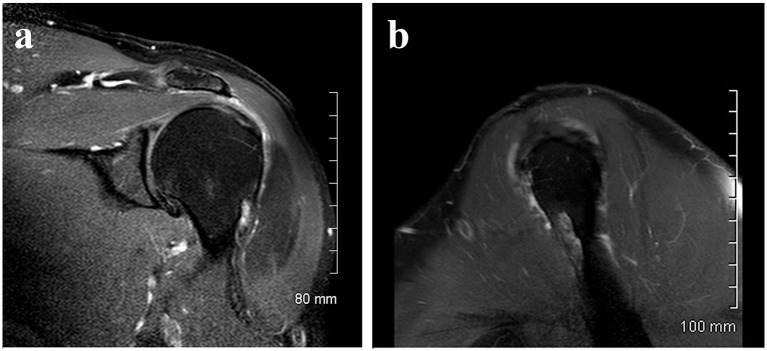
(a) MRI small geode under the cartilage of the humeral. (b) MRI small geode under the cartilage of the humeral.
